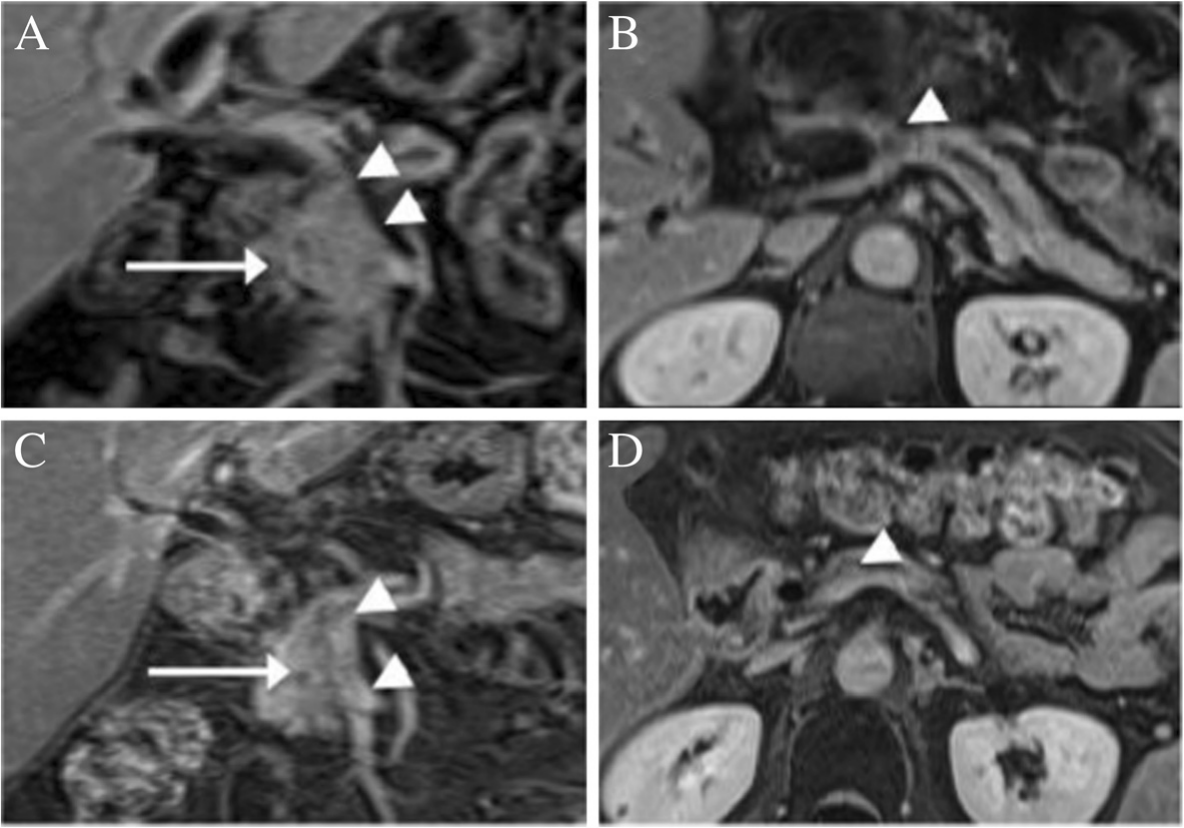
MRI of solid tumor at the pancreatic neck in Case 1. (A,B) Initial MRI, showing a hypovascular pancreatic tumor compatible with adenocarcinoma (arrow). The tumor is encasing the porto-mesenteric confluence (arrowheads); (C,D) MRI after neoadjuvant therapy. It shows a regression in the axial diameter of the tumor (arrow) and patency of the PV and SMV (arrowheads).
